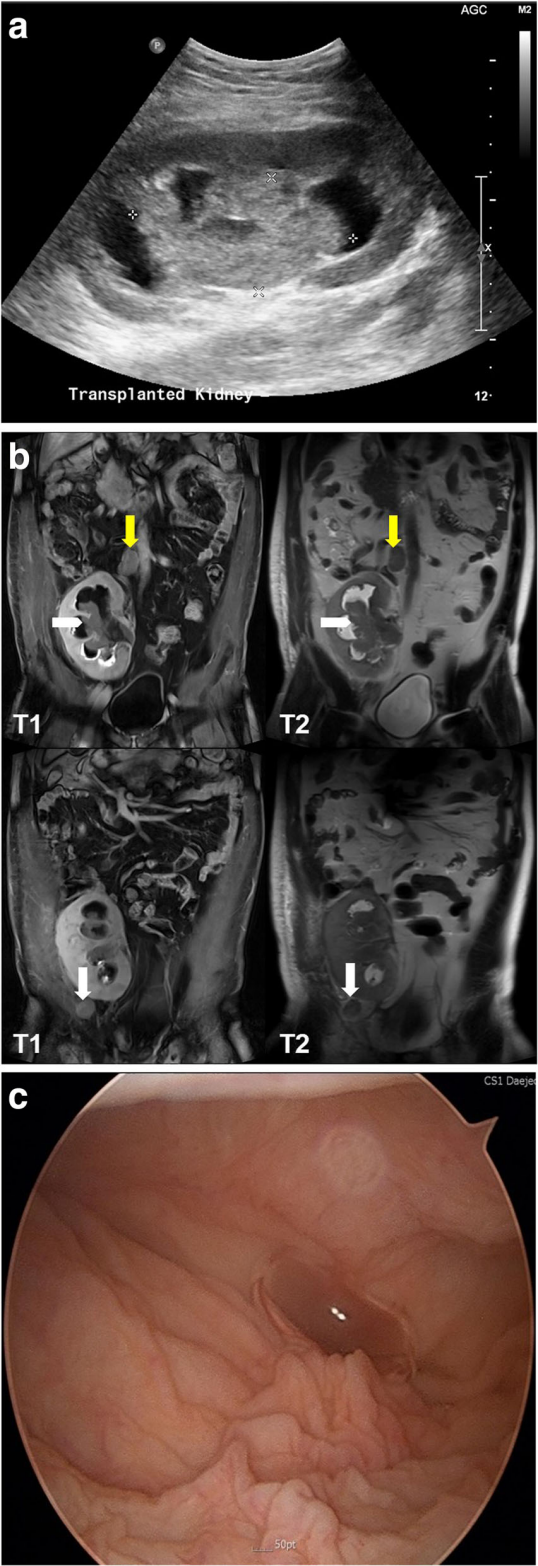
Radiologic examinations and cystoscopic finding in the pelvis mass of graft kidney in a kidney transplantation recipient. a Abdominal ultrasonography shows a huge hyperechoic mass (asterisks) in the pelvis of graft kidney. b Abdominal magnetic resonance image shows a huge polygonal mass filled within whole pelvis of graft kidney (white arrowhead), a 3.3 cm sized mass in aortocaval chain (yellow arrow) and a 2.5 cm sized nodule in right external iliac chain (white arrow) in coronal view. (Left column: T1 weighted image with gadolinium enhancement, Right column: T2 weighted image) c Cystoscopy shows normal graft ureter orifice and normal bladder mucosa
